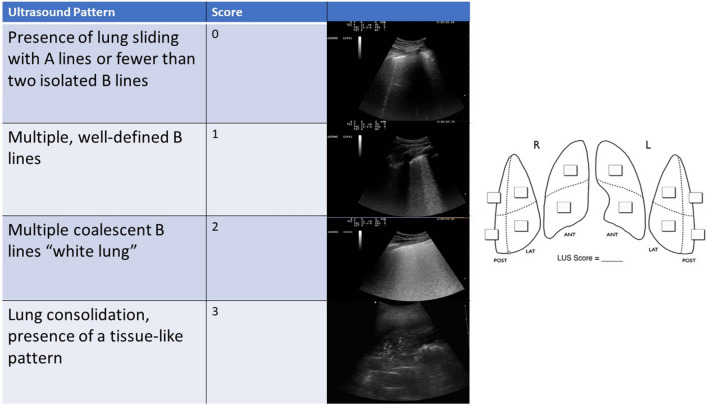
Lung ultrasound score execution scheme
